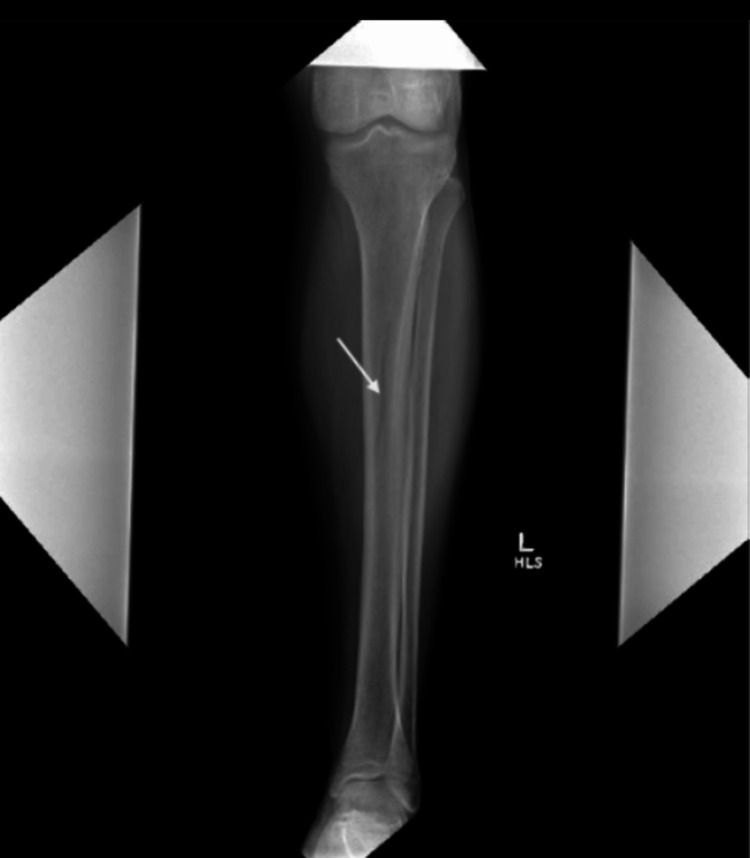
X-ray of the left tibia showing a subtle shadow in the mid tibial shaft; no fractures are present
